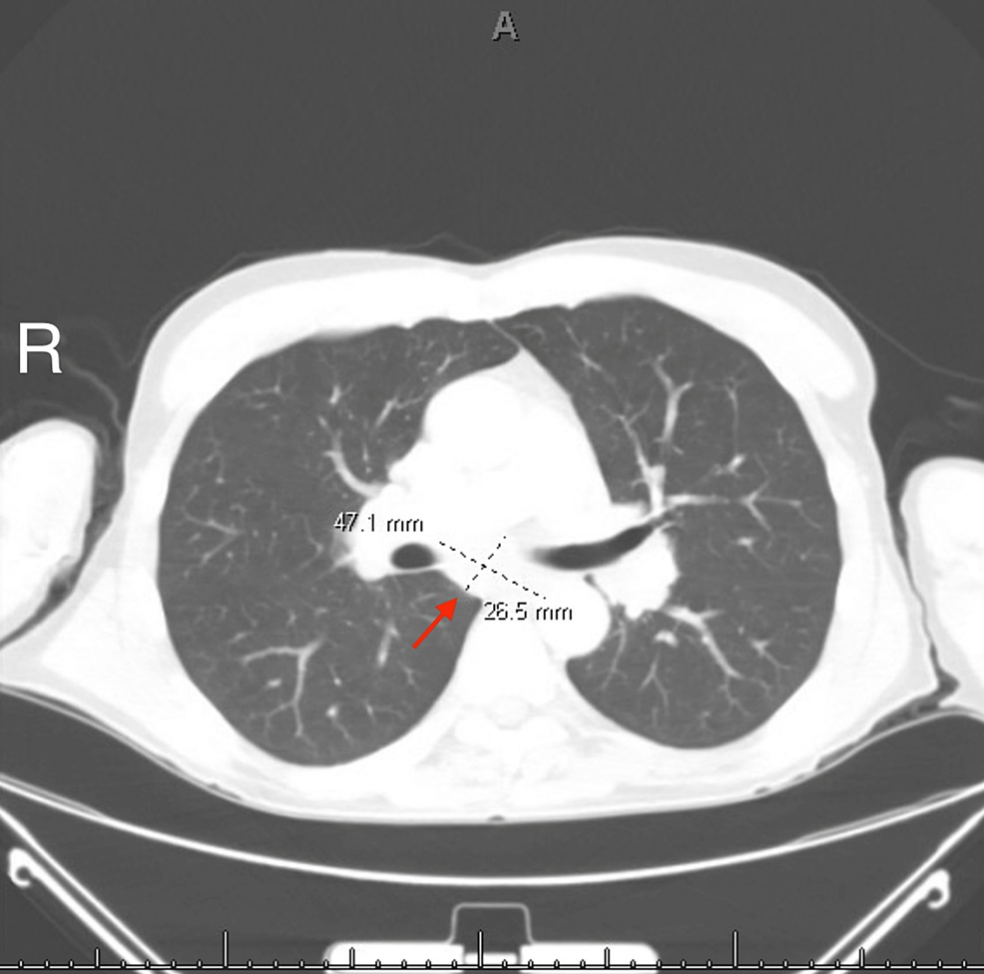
CT of the chest depicting an enlarged subcarinal lymph node measuring 4.7 cm x 2.7 cm. CT: computed tomography.
